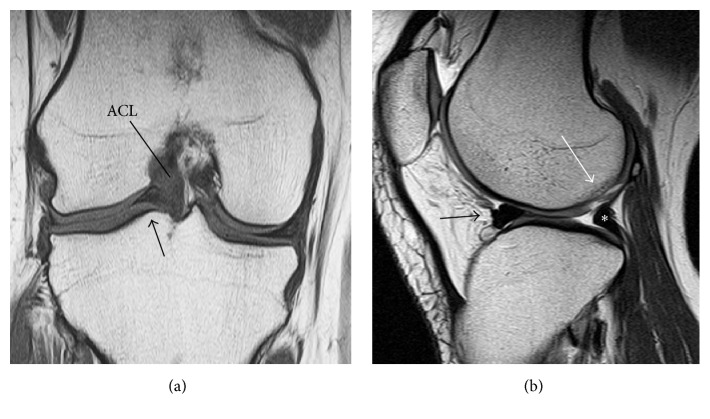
Magnetic resonance imaging of the right knee joint. (a) T1-weighted coronal image showing the posterior segment of the lateral meniscus dislocated into the intercondylar space. The arrow indicates the dislocated posterior segment, which is adjacent to the anterior cruciate ligament (ACL). (b) T2-weighted sagittal image of the lateral compartment depicting the middle segment of the lateral meniscus flipped onto the anterior segment, producing the flipped meniscus sign (black arrow). The popliteus tendon is located on the tibial plateau (asterisk) and displays a similar appearance to the posterior segment of the lateral meniscus. The white arrow indicates an osteochondral defect on the lateral femoral condyle.
